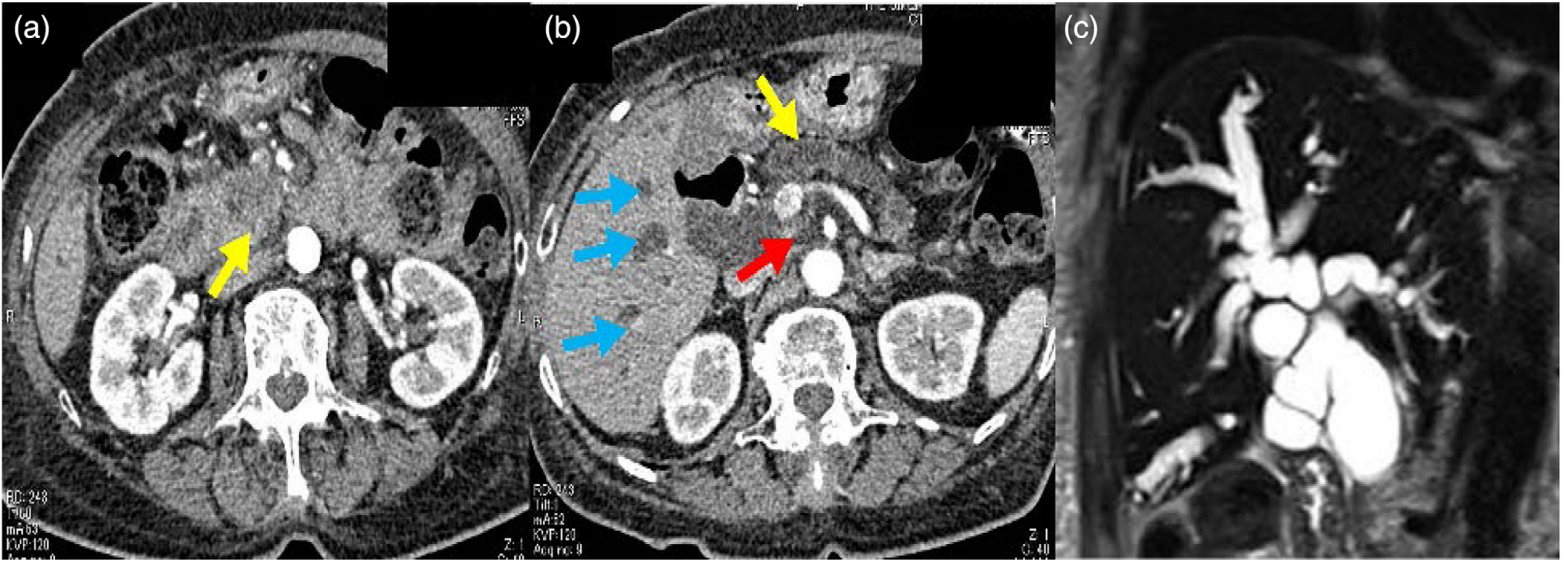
Abdominal contrast computed tomography and magnetic resonance cholangiopancreatography images. (a) Contrast‐enhanced computed tomography arterial phase. A 40‐mm large low‐density area is observed at the head of the pancreas (arrow). (b) Contrast‐enhanced computed tomography. Infiltration of the celiac and superior mesenteric arteries is observed (yellow arrow). The main pancreatic duct is dilated (red arrow). Multiple hepatic metastases are revealed (blue arrow); however, there are no significant lymph node metastases. (c) Magnetic resonance cholangiopancreatography showing dilatation of the bile duct and main pancreatic duct
